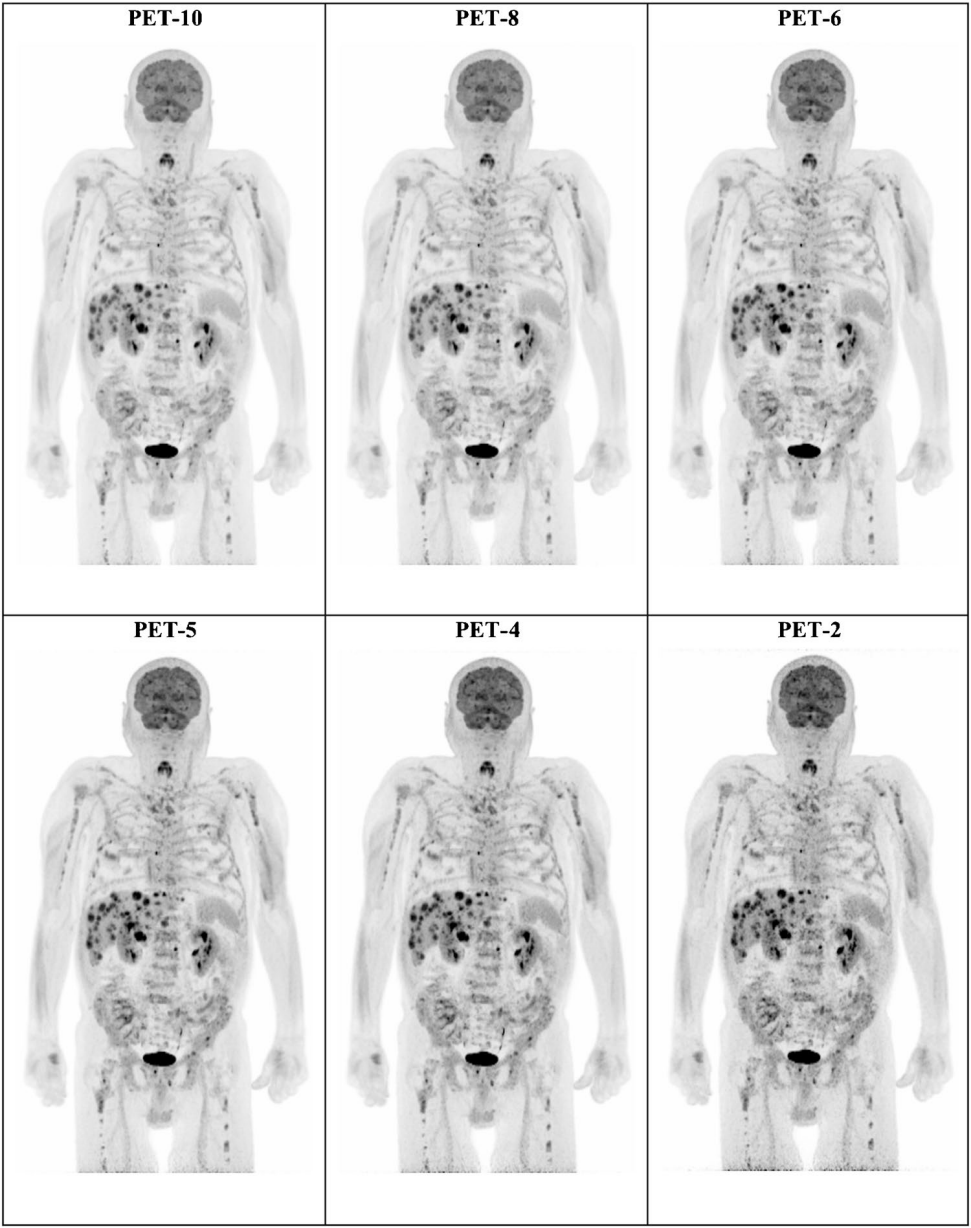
Example of maximum intensity projection (MIP) [^18^F]FDG PET images of a 74-year-old man with stage IV cutaneous melanoma planned for treatment with immune checkpoint inhibitors. Presented are the PET-10, PET-8, PET-6, PET-5, PET-4, and PET-2 acquisitions. All acquisitions clearly demonstrate disseminated [^18^F]FDG-avid metastastic disease
